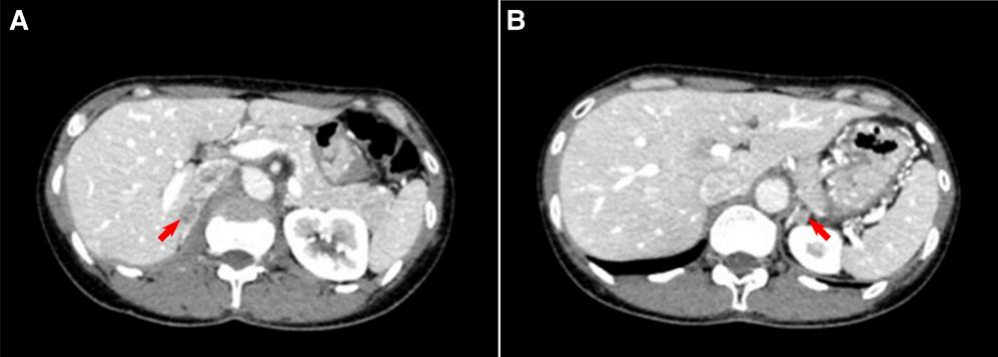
Results of adrenal computed tomography. (A and B) Representative images of contrast-enhanced adrenal CT scan. Red arrows indicate adrenal tumors (A, Right adrenal tumor. B, Left adrenal tumor). CT, computed tomography.
